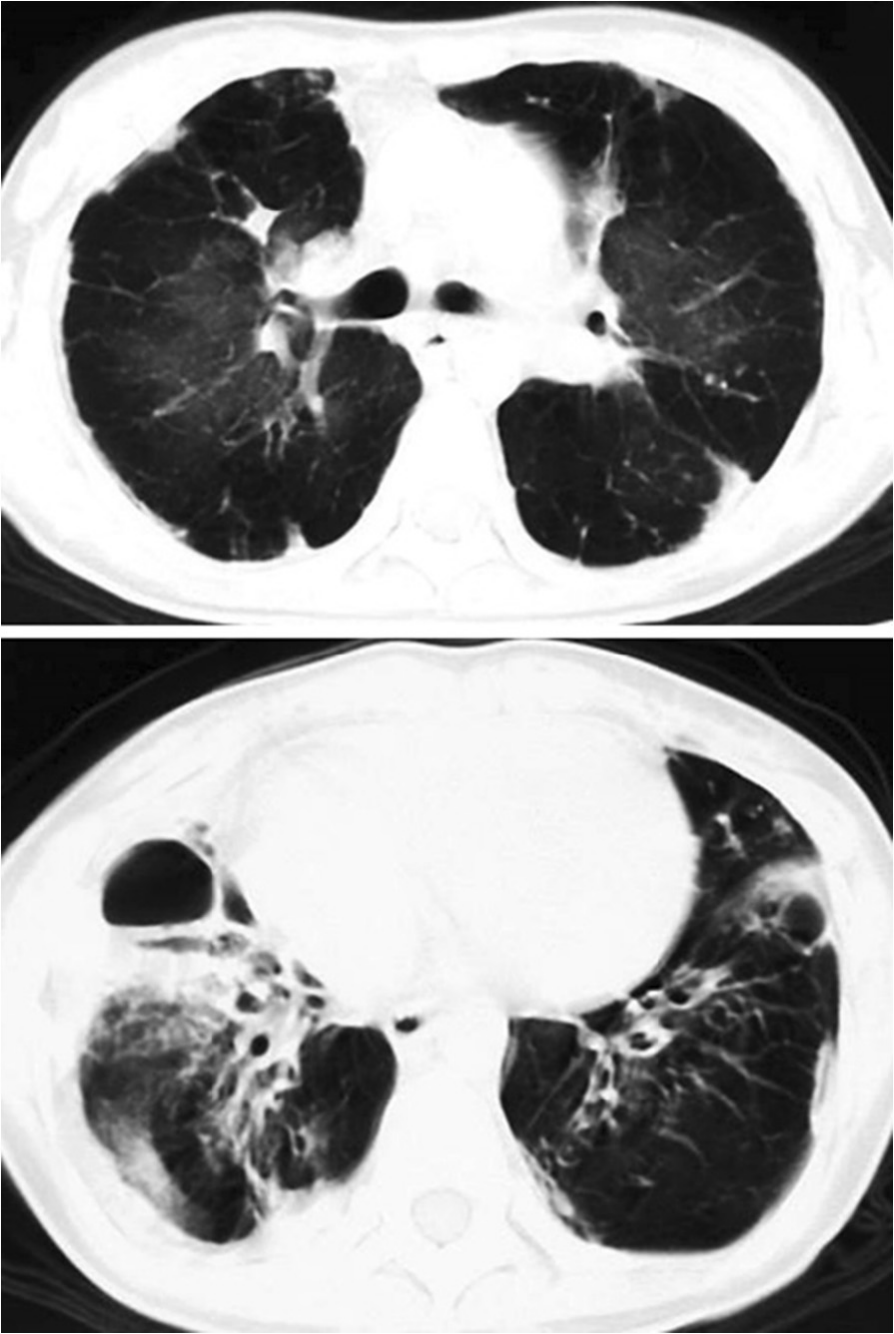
Chest CT scan revealed a widespread mosaic pattern with air trapping and diffuse pleural thickening in both lungs and prominent broncho-bronchiolectasis in the bilateral lower lobes predominance.
